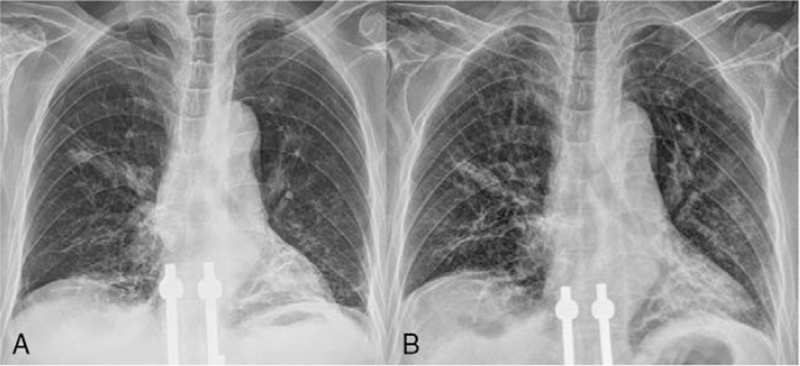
Chest radiographs taken (A) at the end of nontuberculous mycobacteria treatment; and (B) 1 year after the end of treatment.
